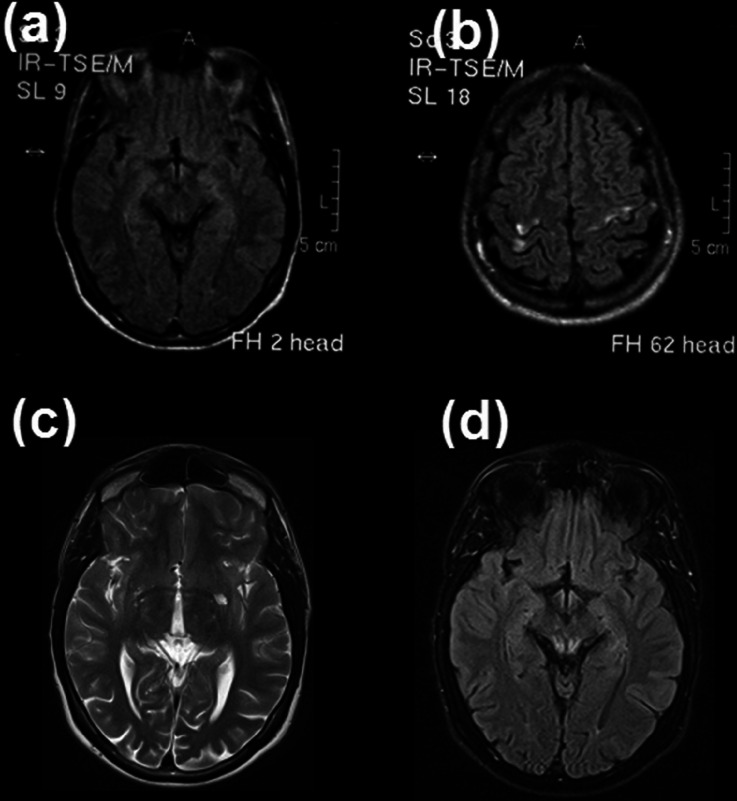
MRI T2 and Flair. Images (a) and (b) performed at the age of 22. (a) Hyperintensive lesion in right cerebral peduncle (b) hyperintensive cortical lesions in the frontal and parietal lobes bilaterally. Images (c) and (d) performed at the age of 26. (c) Hyperintensive lesion in left thalamus (d) symetrical dorsal hyperintensities of brainstem
